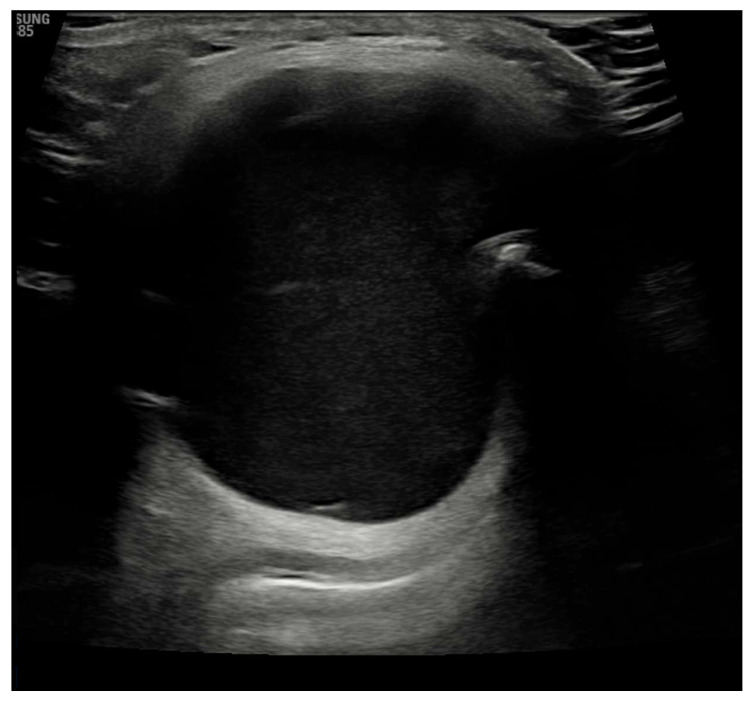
Ultrasound image showing echo-normal and homogeneous thyroid gland.
